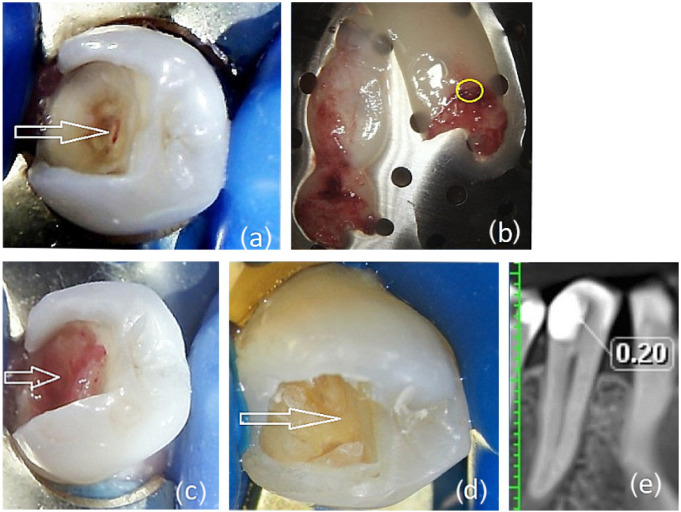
Forty-five-year-old patient with reversible pulpitis treated by direct pulp capping. (a) After removing the infected dentin, a pulp exposure communicating with the axial wall was visible (white arrow). (b) Prepared A-PRF+ membrane; the yellow circle marks the site within the buffy coat from which the material was harvested. (c) The exposure was covered with an A-PRF+ membrane placed on the axial wall of the cavity (white arrow). (d) After ninety days, the temporary filling was removed. The white arrow shows no communication. (e) CBCT image of tooth 45 after restoration 90 days after beginning treatment. There is no communication, as indicated by the white arrow. The reparative dentin had a thickness of 0.2 mm.
